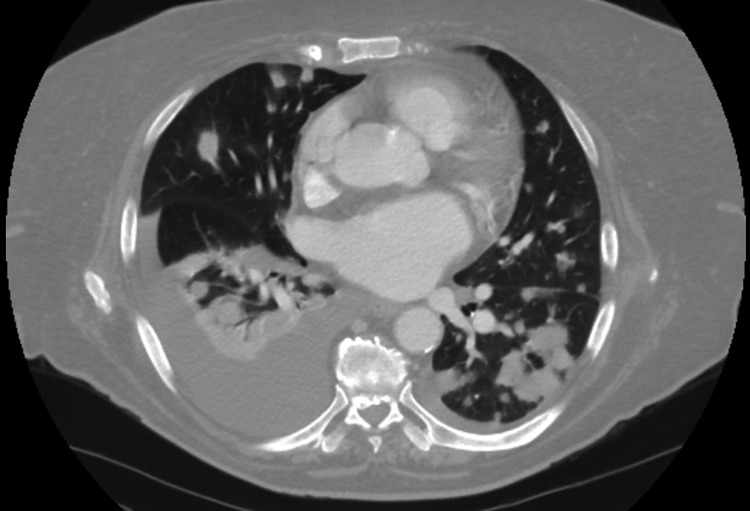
CT chest with contrast demonstrating multiple areas of rounded infiltrates with air bronchograms, mediastinal adenopathy, and right pleural effusion
